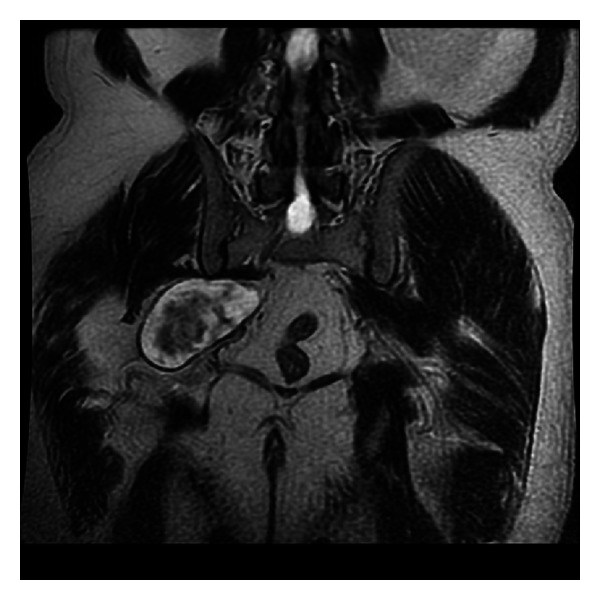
Coronal MRI of the lesion.
